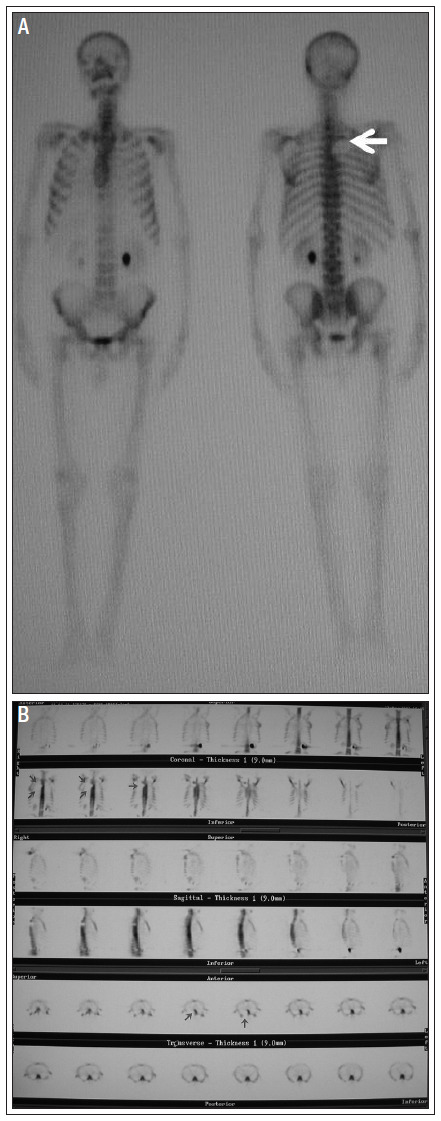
Whole body bone scan (A) in single-photon emission-computed tomography mode (B), revealing areas of decreased radiotracer uptake in the T3-T5 vertebrae and posterior arch of the third to fifth right ribs.
